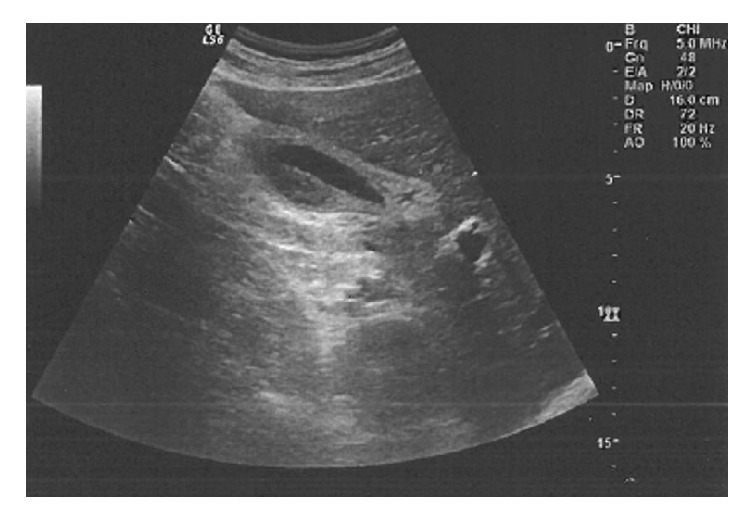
Abdominal ultrasound showing pericholecystic fluid collection, large free fluid at Douglas's pouch, and right paracolic gutter.
